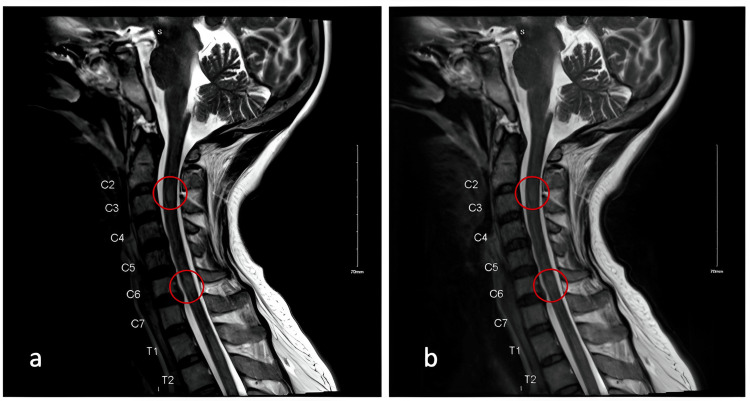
MRI T2-FLAIR cervical spine sagittal view with and without IV contrast. Moderate multilevel degenerative changes. Areas of T2-FLAIR hyperintensity posterior to C2 and C6 vertebral bodies (a, b)
